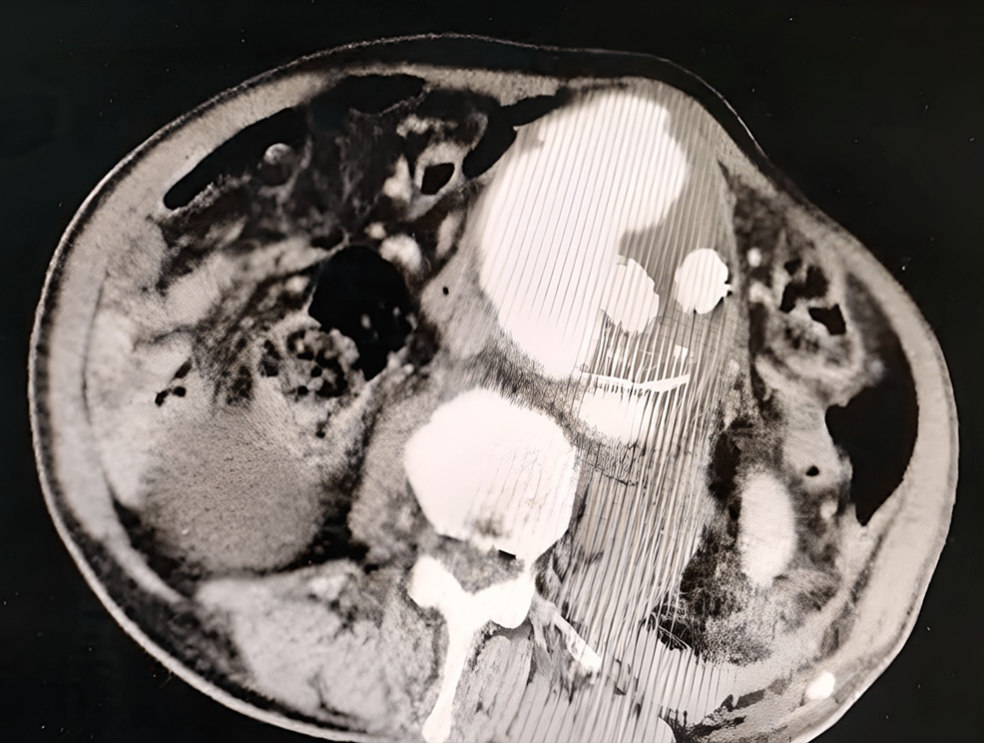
Axial slice of CT angiography demonstrating an endoleak with aortic rupture and hemoperitoneum.
